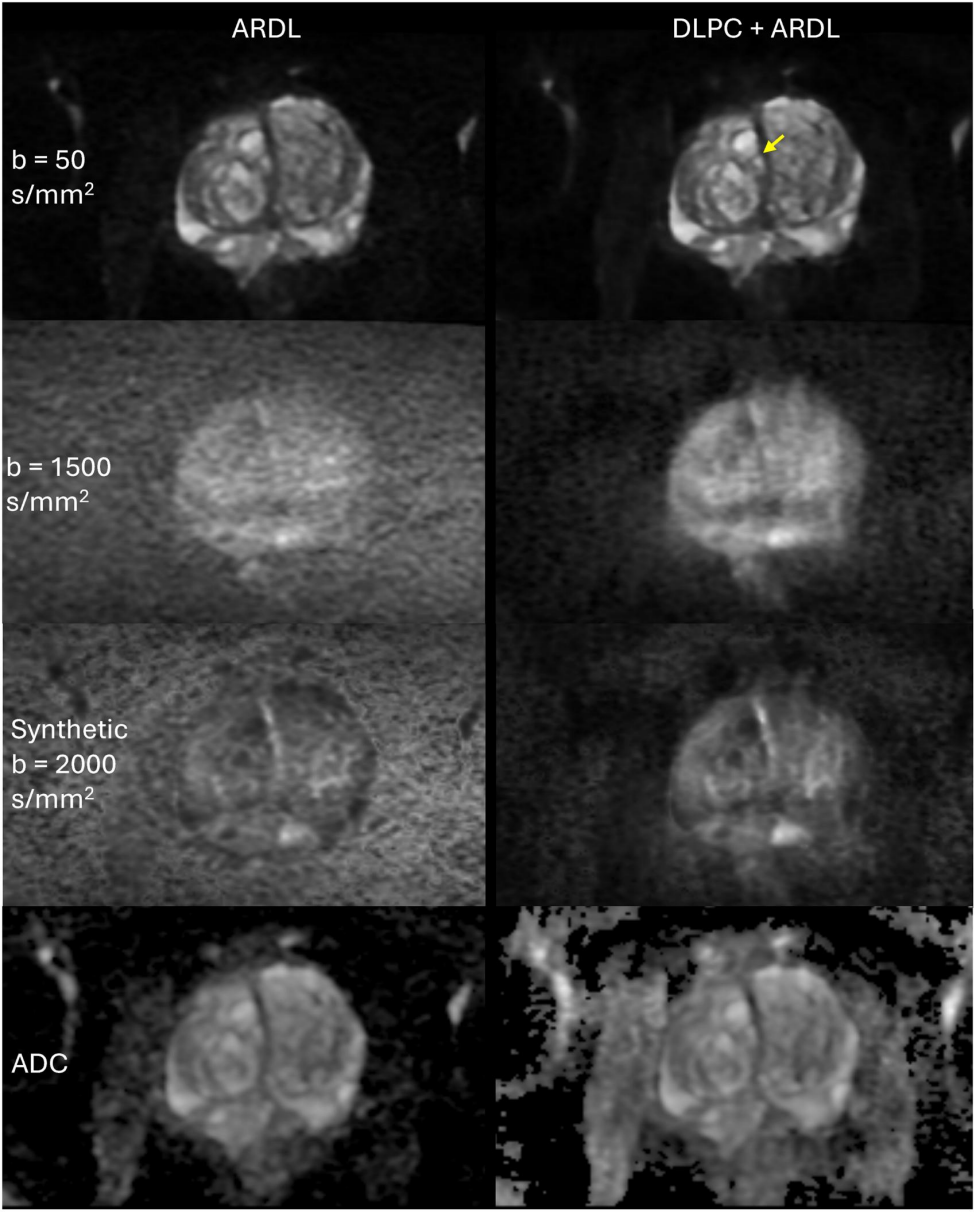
Diffusion-weighted images (DWI) acquired using the 50-channel pelvic coil (first and second row) reconstructed with product AIR Recon DL (ARDL) denoising (left) and DL Phase Correction with ARDL (DLPC + ARDL) (right), the corresponding synthetic *b = *2000 s/mm^2^ images (third row), and apparent diffusion coefficient (ADC) maps (bottom row) are shown with matched window and level between the image pairs. Compared to the improvements in Figure 2, the improvement resulting from DLPC reconstruction is much more significant. At *b = *50 s/mm^2^ (top row), small structural details show noticeable improvements in using DLPC+ARDL, such as a better delineation of the border of the benign nodules (yellow arrow). DLPC+ARDL reconstructed images have strong background noise suppression, particularly noticeable at *b = *1500 s/mm^2^ (second row), leading to a better delineation of the prostate gland and distinctive hyperintense lesion border. Improved image quality with DLPC reconstruction results in improved ADC estimation, evident in clear prostate gland delineation and clear border visualization in hypointense signal regions signifying restricted diffusion, and more homogenous signals in low SNR regions, such as muscles.
